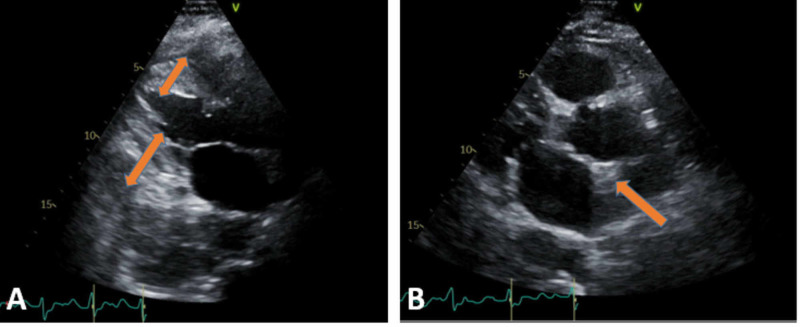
ECHO: (A) TTE in parasternal long-axis view demonstrating concentric LVH and thick IVS, as pointed by the orange arrow, and (B) TTE subcostal view demonstrating concentric LVH. ECHO, echocardiogram; TTE, transthoracic echocardiogram; LVH, left ventricular hypertrophy; IVS, interventricular septum
